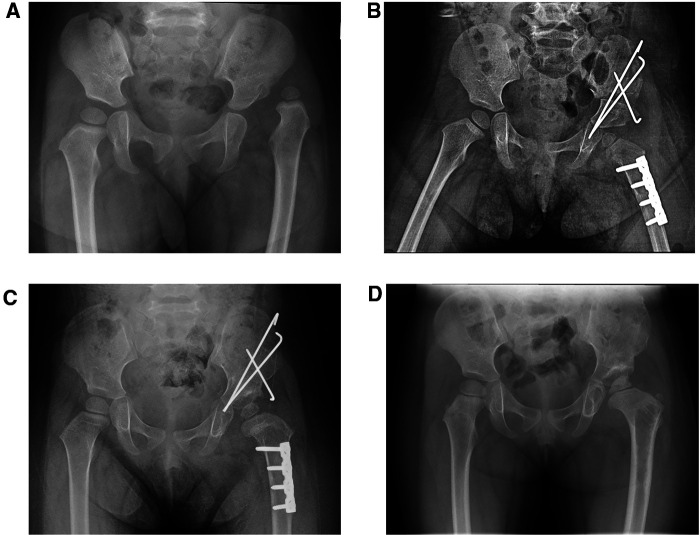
A girl, aged 2 years and 4 months, with Tönnis III dislocation of her left hip, underwent a Salter pelvic osteotomy combined with femoral shortening osteotomy. (A) Preoperative hip anteroposterior (AP) radiograph: left hip dislocation. (B) AP radiograph obtained one month after the Salter pelvic osteotomy combined with femoral shortening osteotomy. The length of the femur osteotomy was 1.5 cm. (C) AP radiograph obtained six months after the Salter pelvic osteotomy combined with femoral shortening osteotomy. (D) AP radiograph obtained 14 months after the Salter pelvic osteotomy combined with femoral shortening osteotomy and 6 months after removal of internal fixation.
